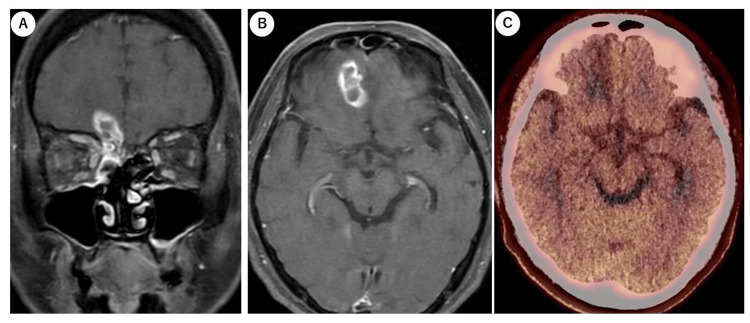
Final MRI and MET PET imaging A, B: coronal and axial MRI images. The most recent MRI findings indicated no lesions in the right paranasal cavity; however, the space-occupying lesion (SOL) in the cranial cavity showed increased contrast enhancement in some areas, and the edematous changes in the surrounding brain parenchyma had worsened. C: MET PET imaging. Mean standardized uptake (SUVmean) of 1.39 in the tumor and 1.07 in normal brain tissue was observed. MET: 11C-methionine; PET: positron emission tomography
